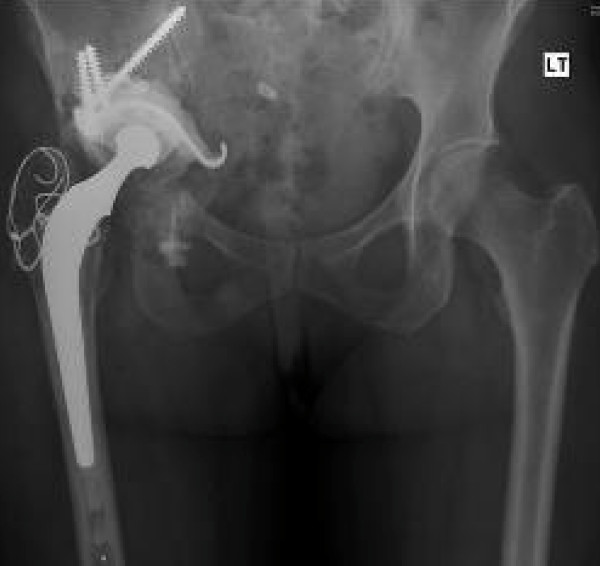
Radiograph showing loose acetabular component 80 months after index surgery.
